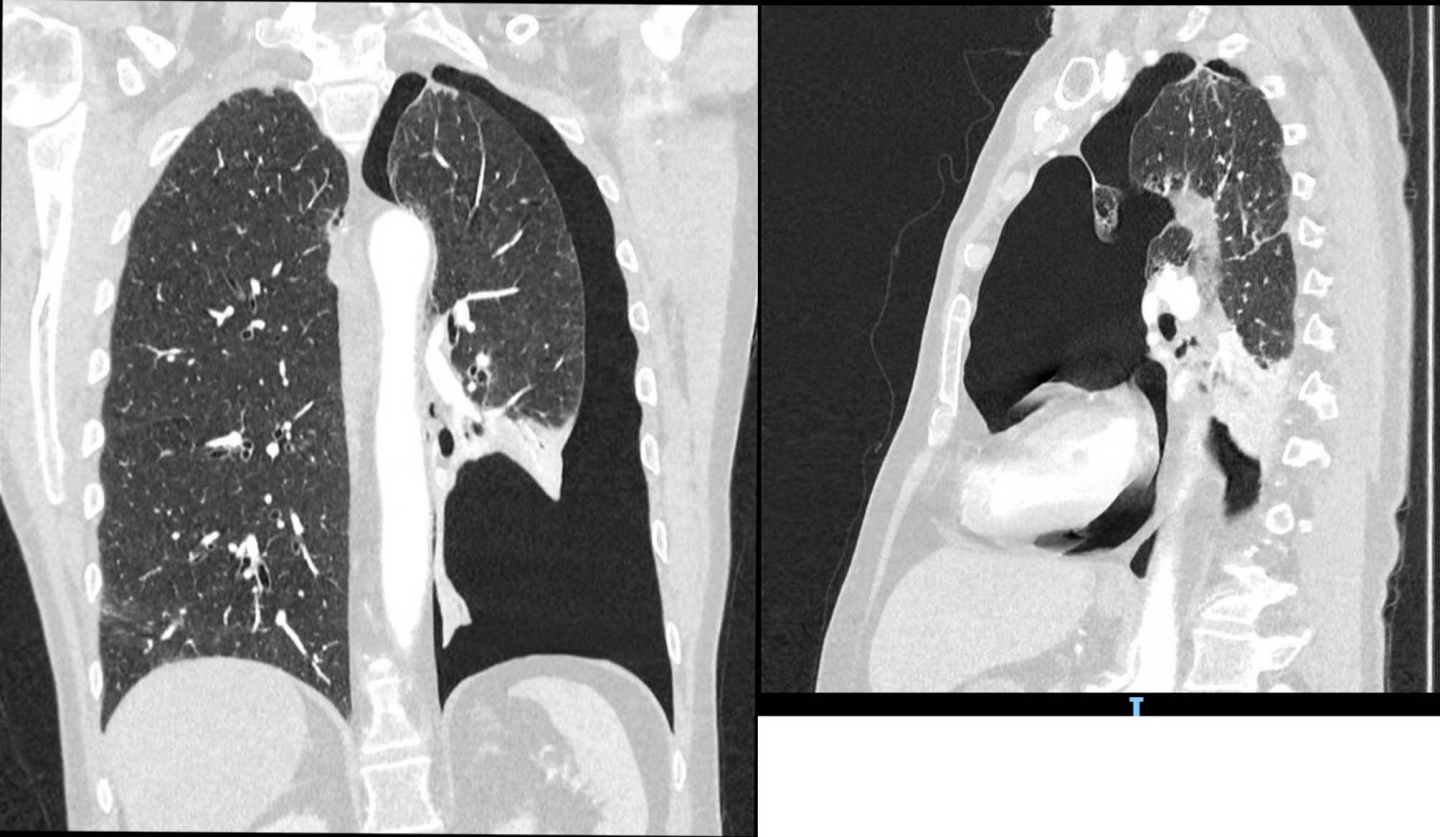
Shows coronal (left) and sagittal (right) views of the CT thorax taken on the patients second admission. A large left-sided pneumothorax can be appreciated
